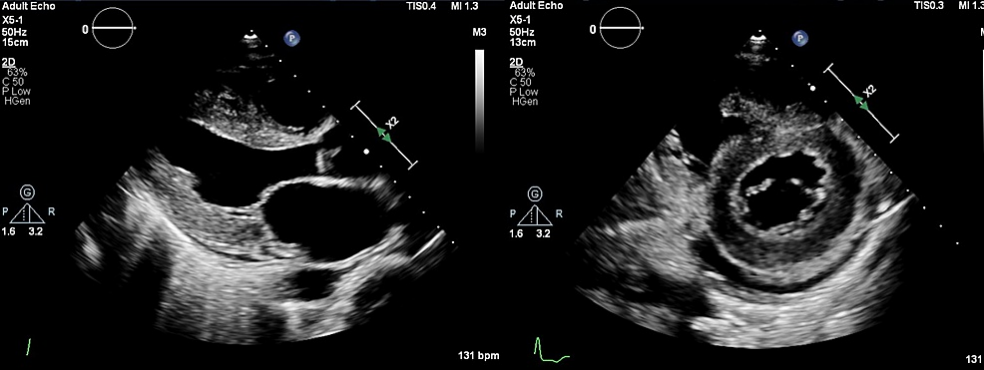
Left side: Parasternal long-axis view of the echocardiogram showing the dilated left atrium and left ventricle; right side: parasternal short-axis view at papillary muscle level showing dilated left ventricle and minimal pericardial effusion (October 20, 2021).
